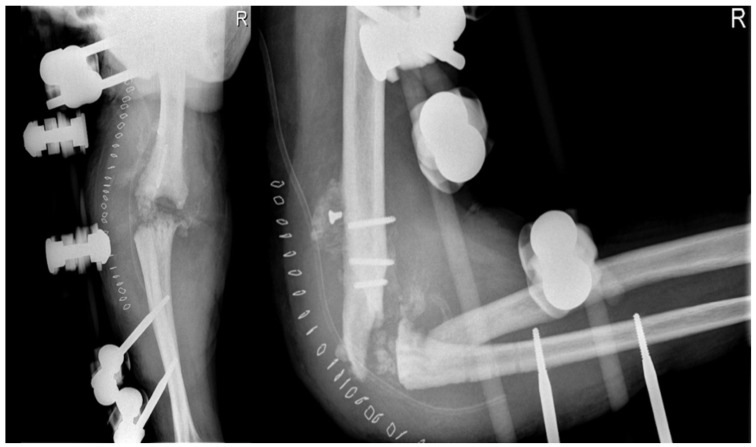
X-ray anteroposterior and lateral views of the right elbow after the External Fixator positioning to stabilize the elbow joint.
